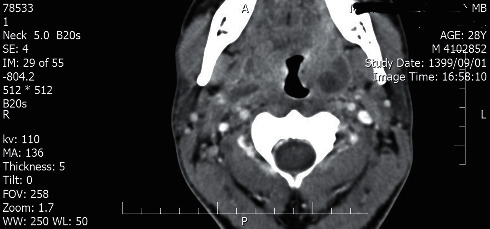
Head and neck CT scan with IV contrast shows left peritonsillar abscess (measuring 14 × 17 mm).
